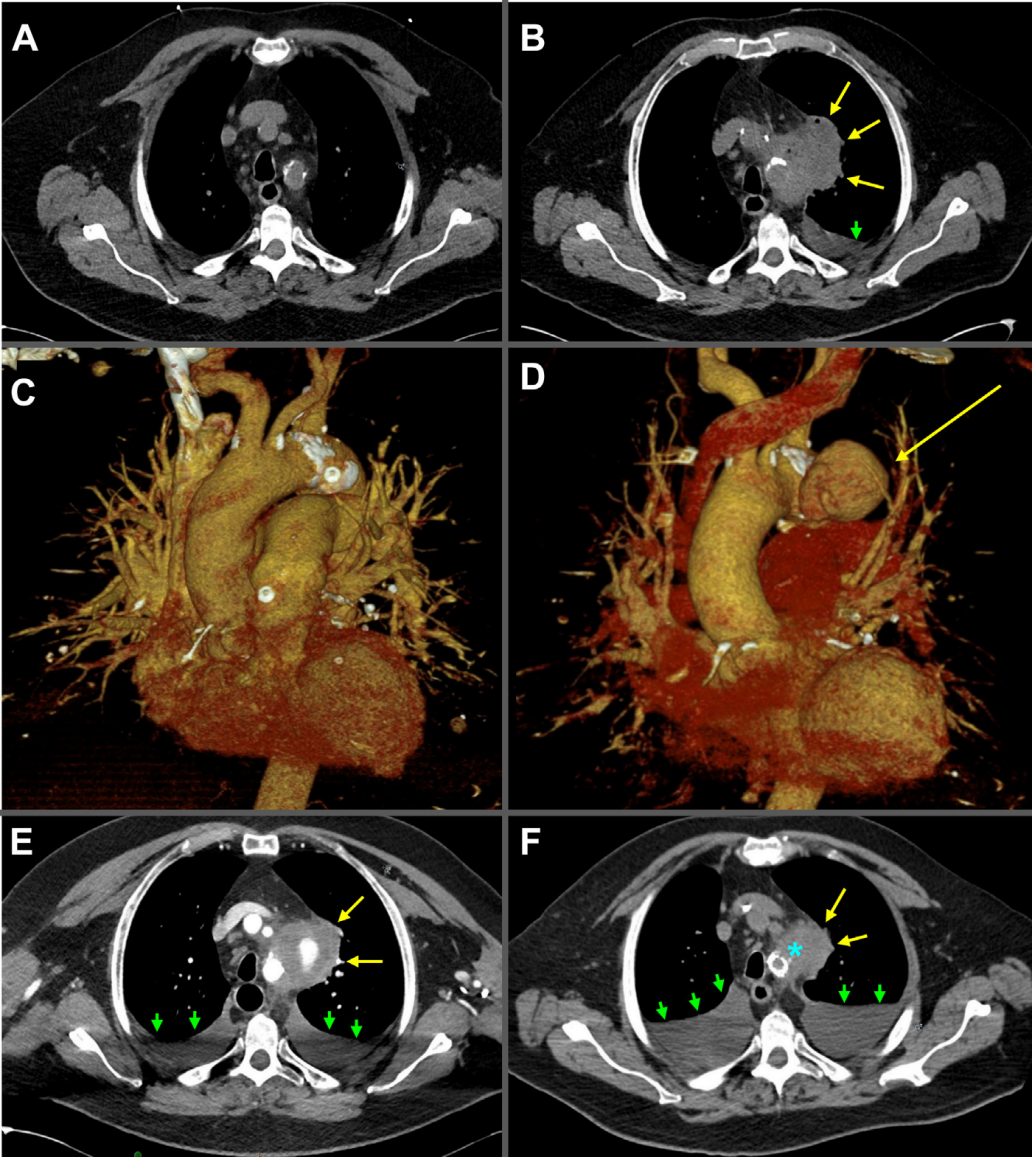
CT Cross-Sectional Imaging at Thoracic Vertebral Level 3/4 and CTA 3D Reconstructions, Demonstrating Radiographic Appearance and Rapid Evolution of the Aneurysm (A, C) Initial images with no aneurysm seen. (C, D) Two months, later showing the “lung mass” abutting the left mediastinum and a left pleural effusion. (E) At 3 months (before TEVAR), showing aneurysmal progression and bilateral pleural effusions. (F) At 6 months (after TEVAR) during an admission for heart failure exacerbation, showing aneurysm stability and progressive bilateral pleural effusions. Yellow arrows point to the saccular aneurysm of the proximal descending thoracic aorta. Green arrowheads point to pleural effusions. Blue asterisk indicates TEVAR. CT = computed tomography; CTA = computed tomography angiography; TEVAR = thoracic endovascular aortic repair.
